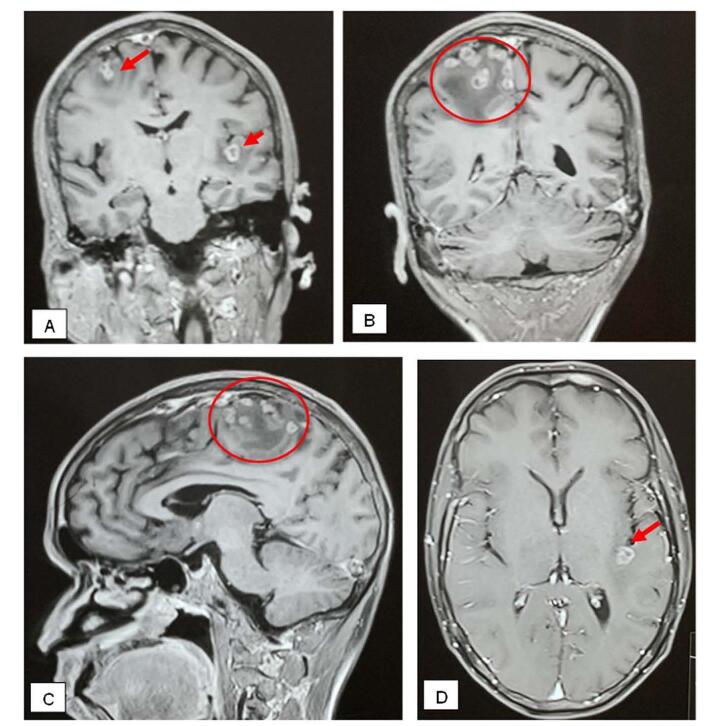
T1 weighted MRI of brain: (A & B) oblique coronal cut, (C) sagittal cut, and (D) axial cut showing features of multiple tuberculomas with associated edema in cerebrum (arrows and rings).
